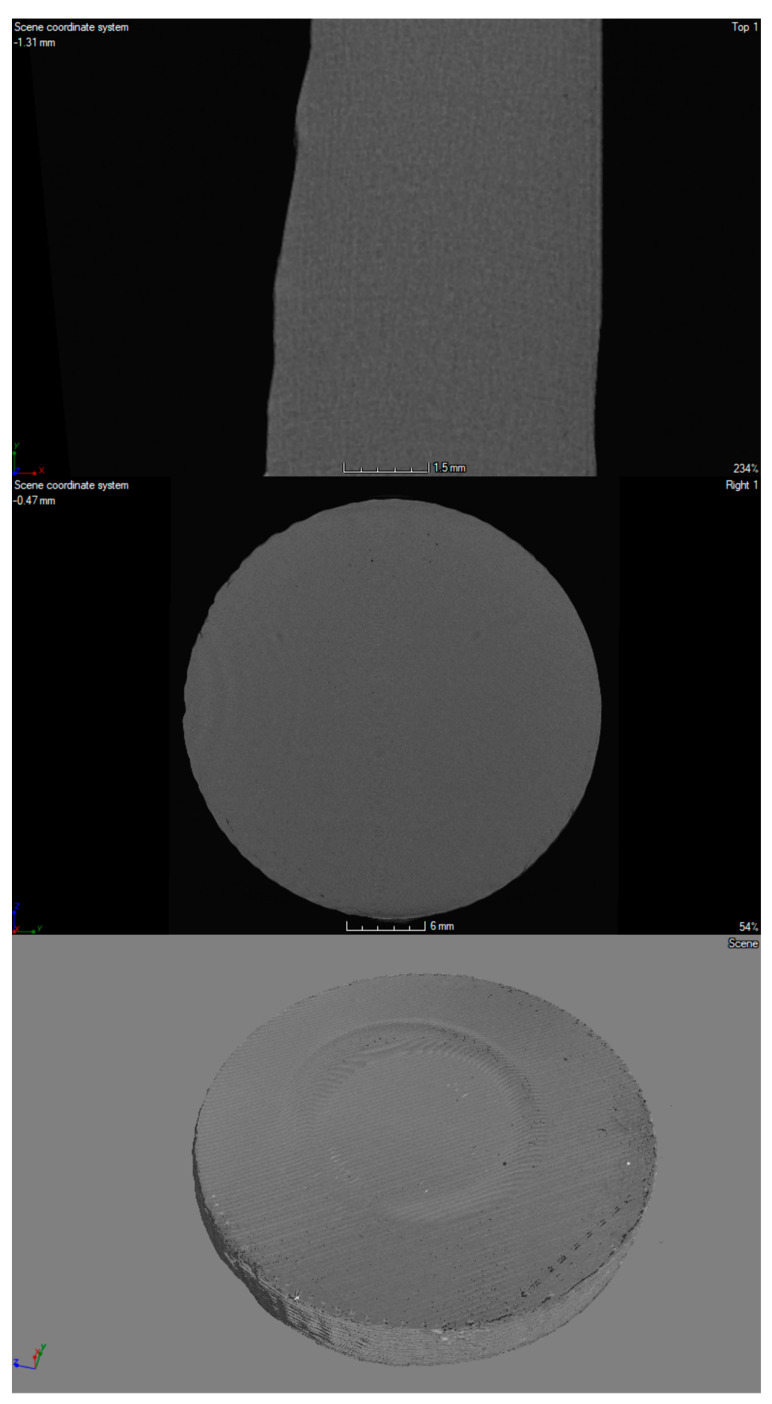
Micro CT imaging of 3D-printed porous standard plate modified with nanosilica.
